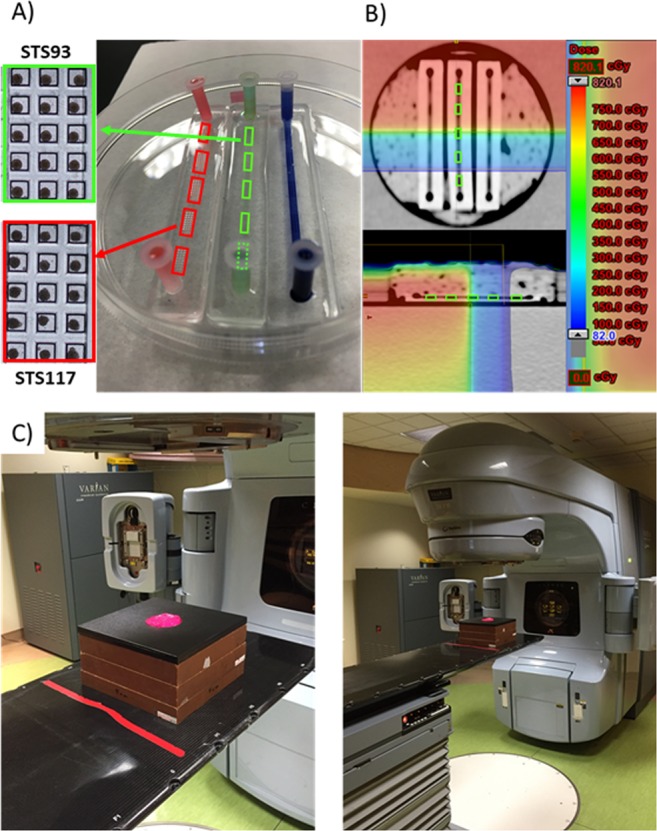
Experimental set-up. Within a 100 mm petri dish, the microfluidic systems are kept in sterile conditions (A). Each spheroid culture well is of the dimension 500 × 500 × 500 µm^3^. Two dozen (24) spheroid wells compose each of the 5 chambers (green and red rectangles), which are linearly connected within a single device. A CT-scan of the devices was done and a radiation dosimetry plan was generated (B) to treat different chambers within the devices with various doses of radiation (color coded) ranging from 0.5 Gy-8 Gy. The device and petri dish is covered by a pink bolus material (C, left), placed and irradiated using a clinical linear accelerator (C, right) as per the radiation plan. Spheroids of STS 93 and STS 117 were formed using the system within (A) 48 and (B) 120 hours following the introduction of a cell suspension of 2 × 10^6^ cells in 1 ml culture medium.
